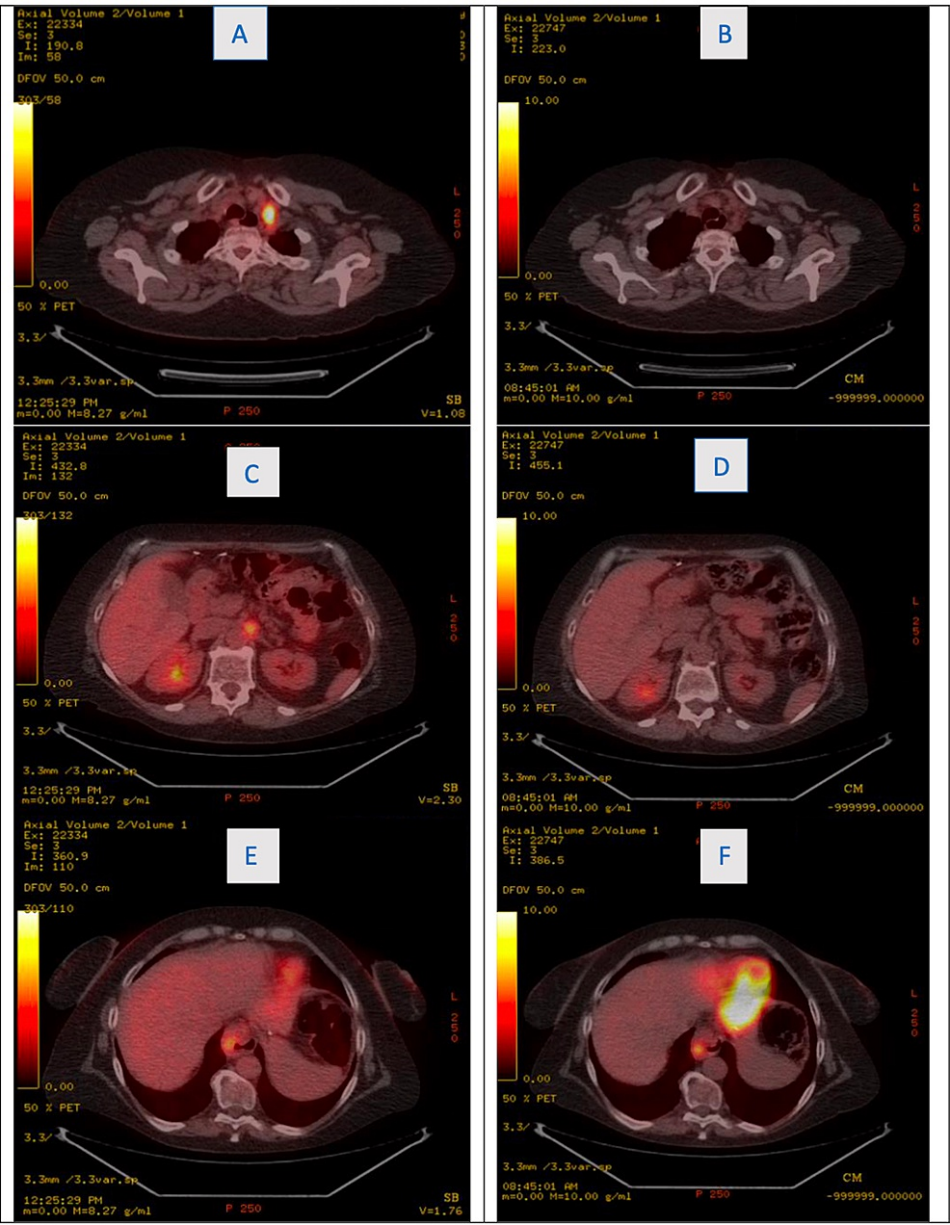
A and B: Interval resolution of supraclavicular lymph node in the neck between December 26, 2020 (A) and April 26, 2021 (B). C and D: Interval resolution of additional para-aortic lymph node in the abdomen between December 26, 2020 (C) and April 26, 2021 (D). E and F: Progression of esophageal mass with interval increase in SUV between December 26, 2020 (E) and April 26, 2021 (F). SUV, standardized uptake value
